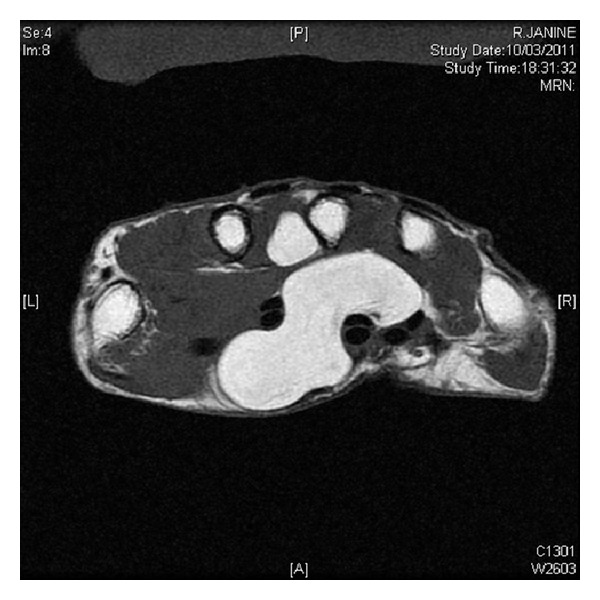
MRI axial view showing the tumor with spontaneous hypersignal rejecting flexors tendons without invasion.
